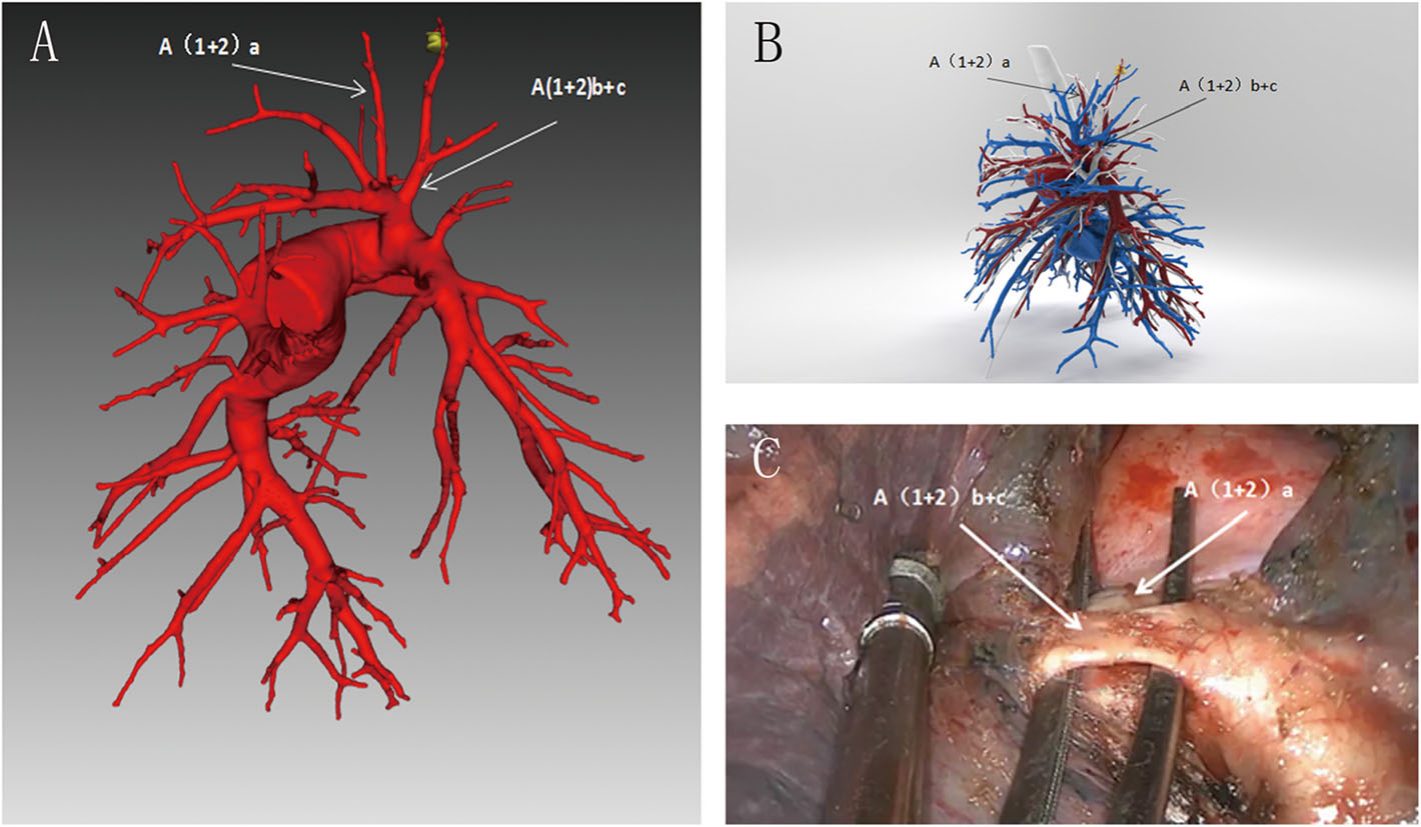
Three-dimensional reconstruction CT image of the same patient and intraoperative-related anatomy. a Image of pulmonary artery reconstruction, with arrows pointing to A(1 + 2)a and A(1 + 2)b + c. b The patient’s pulmonary vein, pulmonary artery, bronchi, and pulmonary nodules are shown in the reconstructed image. c The patient’s intraoperative pulmonary anatomy is consistent with preoperative 3D reconstruction and 3D printing
